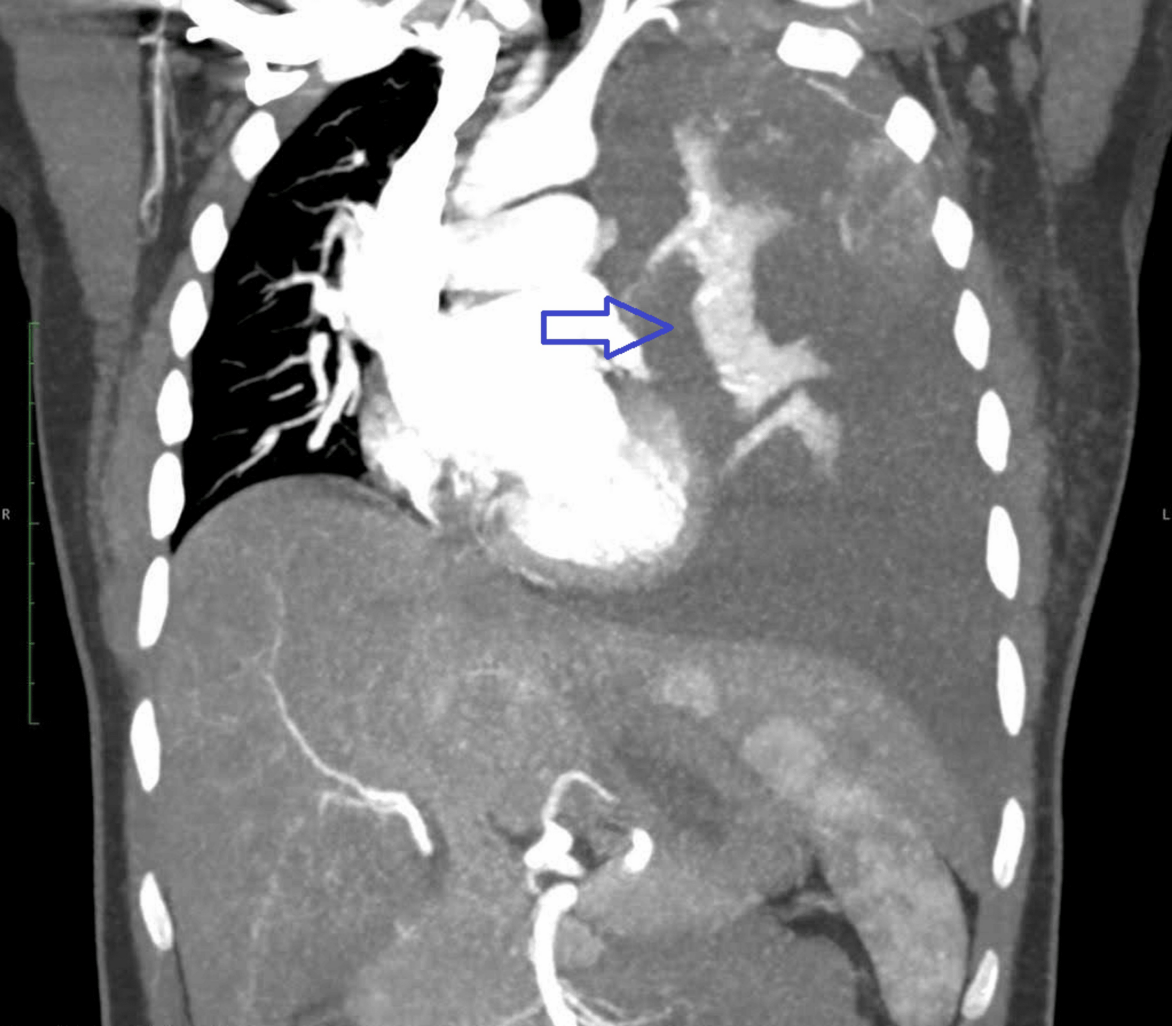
CT angiography CT = Computed tomography
